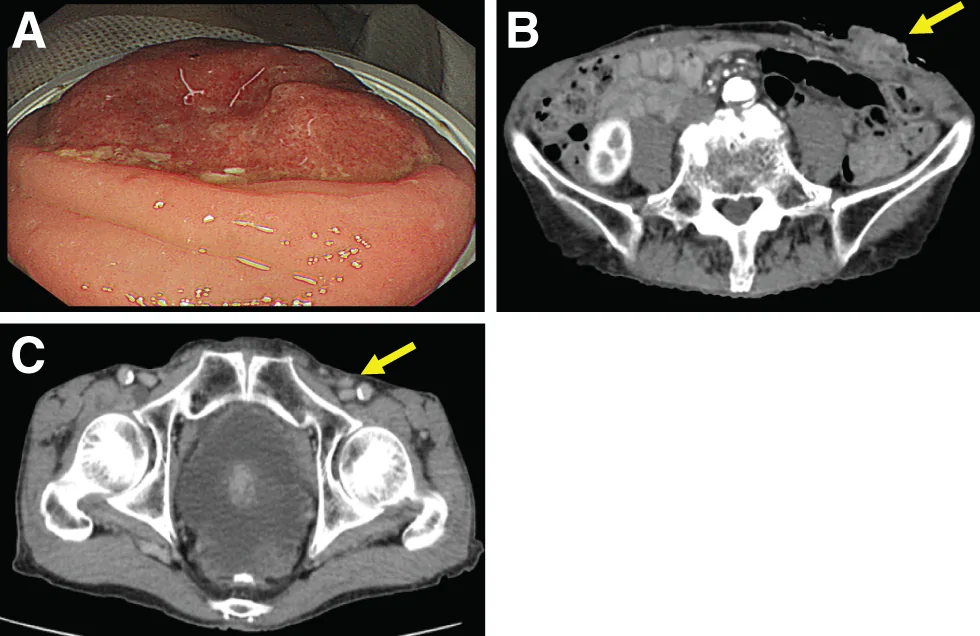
Colonoscopy and contrast-enhanced CT images. (A) Colonoscopy revealed a type 2 tumor at the colostomy site. (B, C) Contrast-enhanced CT revealed hypervascular irregular wall thickening at the stomal site (yellow arrow in B) and a moderately enlarged lymph node in the left groin (yellow arrow in C).
